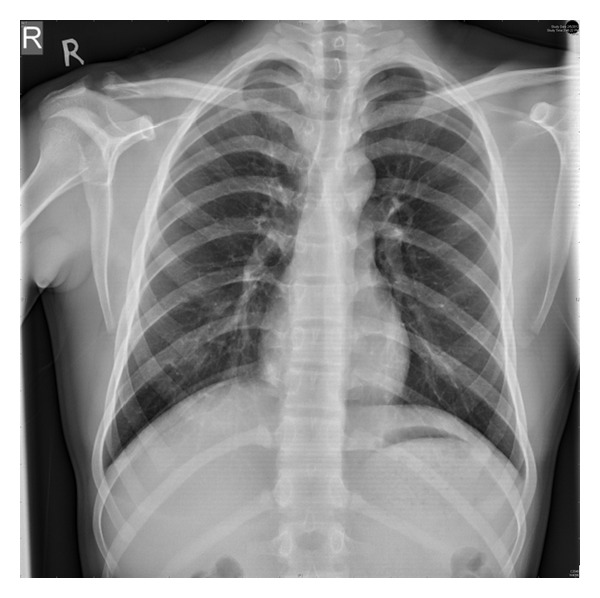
Follow-up radiograph at 18 months showing union at the lateral end of the clavicle and persistent sternoclavicular dislocation.
